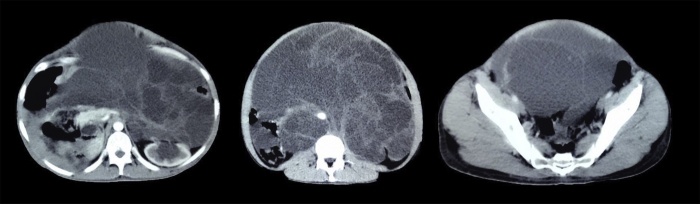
The second CT scan that was made after 3 months of the first operation: showing the aggressive recurrence of the cyst to occupy the abdomen again.
